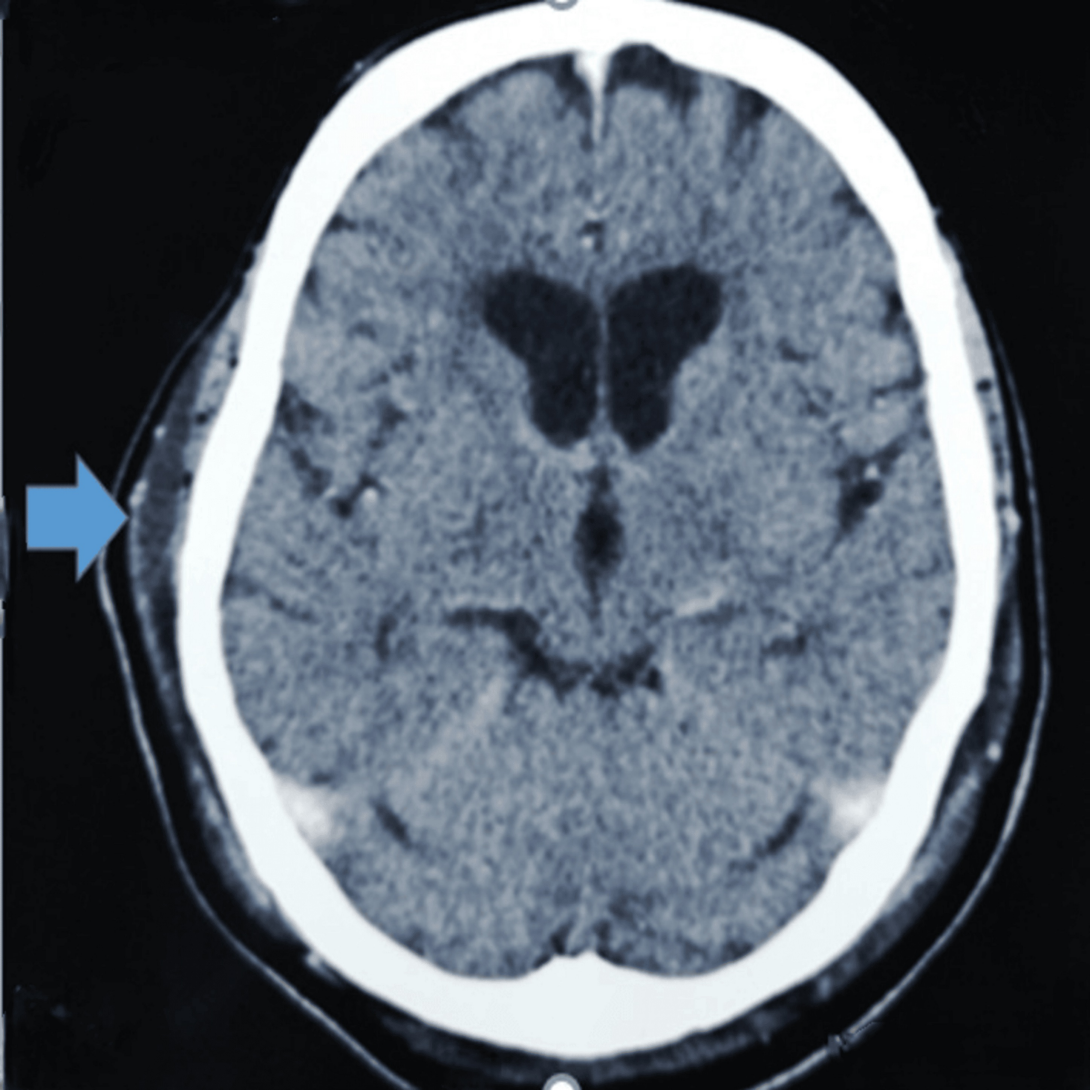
Contrast-enhanced computed tomography scan of the brain showing multifocal old infarcts with right parieto-temporal scalp hematoma (blue arrow).
